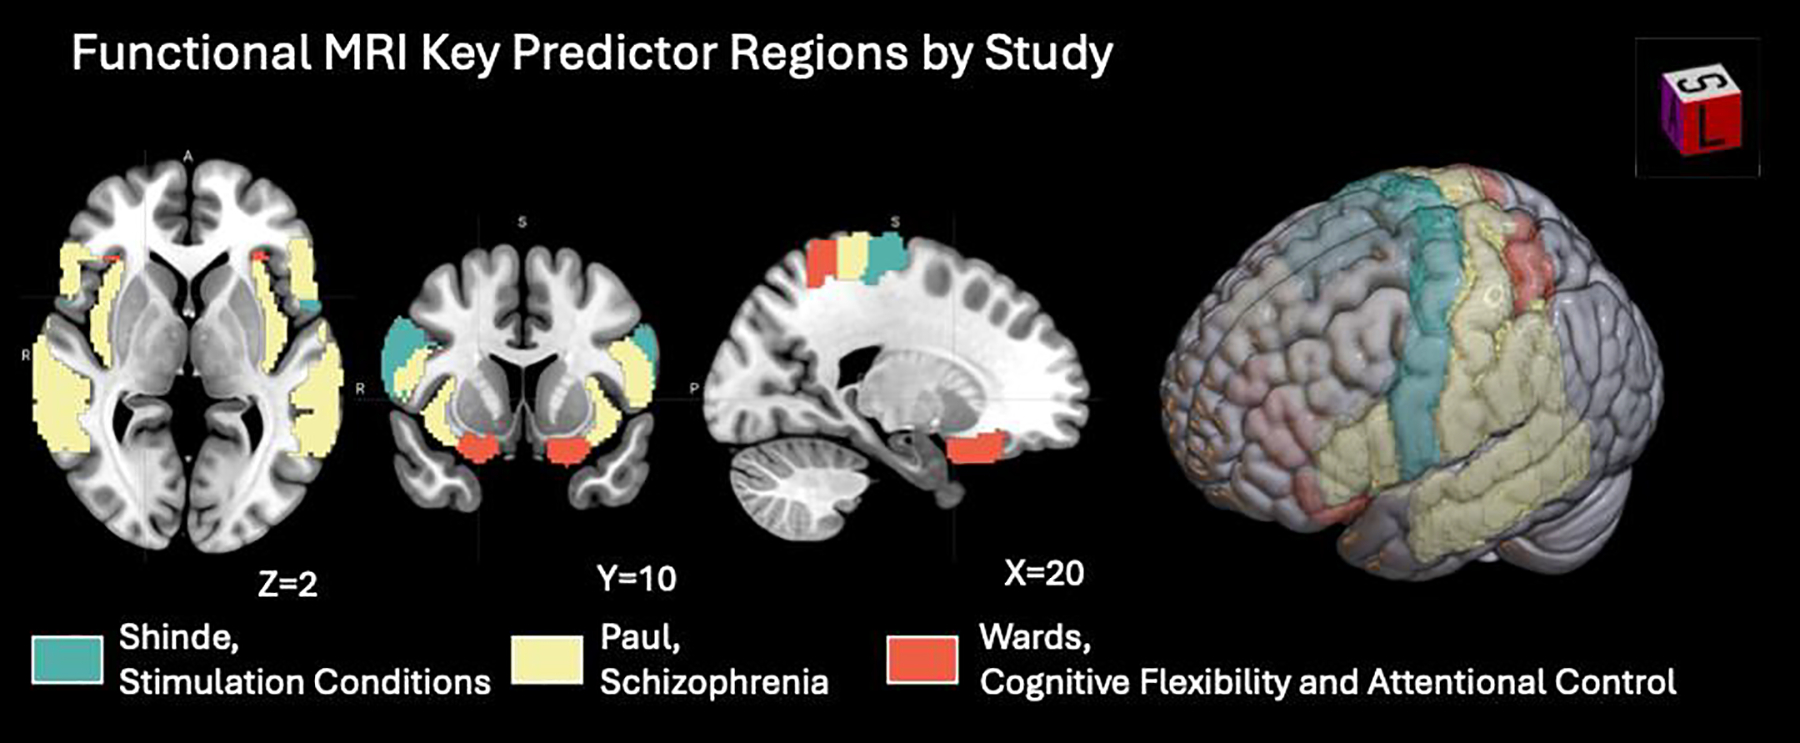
Functional MRI predictor regions across ML studies of tDCS and related therapeutic indications. Visualizations were generated using MRIcroGL v26.0.1 (https://www.nitrc.org/), with overlays mapped onto the MNI152 template supplied by the software.
